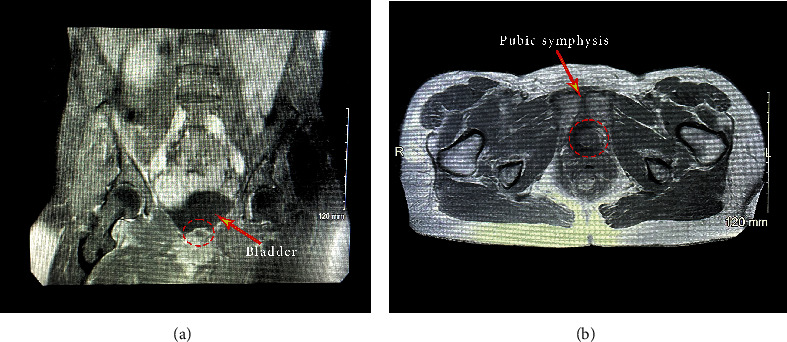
MRI of the pelvis demonstrating an undefined structure in the posterior inferior left paramedian bladder wall protruding into the bladder (red circle). Image demonstrates decreased T1 with minimal increased T2 with questionable minimal enhancement of a thin rim with no internal enhancement.
